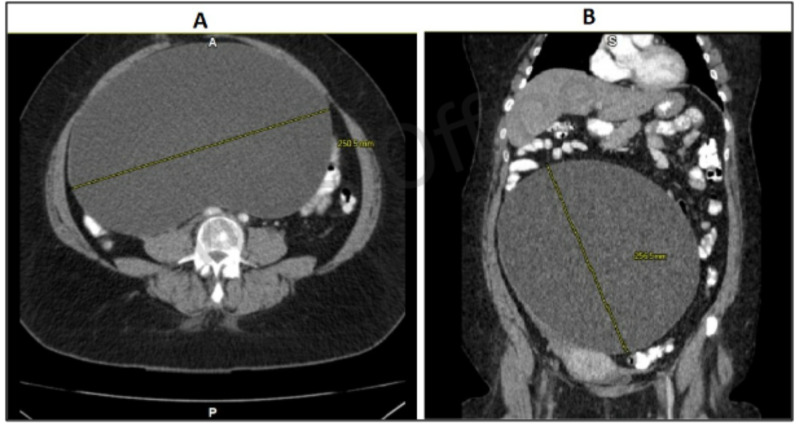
Abdominal CT in axial (A) and coronal (B) sections showing large, well-defined, unilocular cystic mass measuring 25 × 25 cm.
